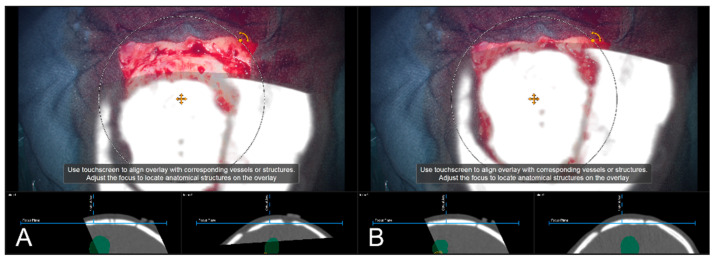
Navigation inaccuracy seen in the recent focus plane utilizing a MIP of the intraoperative automatic registration CT image set acquired after craniotomy showing the translational mismatch of MIP and patient anatomy (A) and the match of MIP and patients anatomy in the recent focus plane after manual correction (translation) of the visual misalignment (B). (In parallel view of MIP projection (upper part) and inline views with the recent focus plane (blue line) and the optical axis (dashed blue line) in the bottom part).
